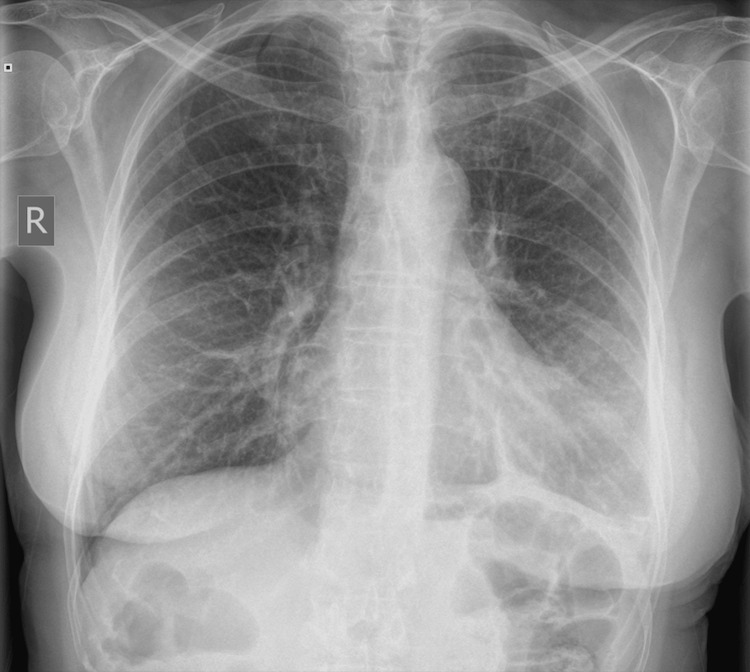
Chest X-ray demonstrating patchy left basal opacification with additional infiltrates in the left upper zone; right lung field clear and cardiomediastinal contours within normal limits.
